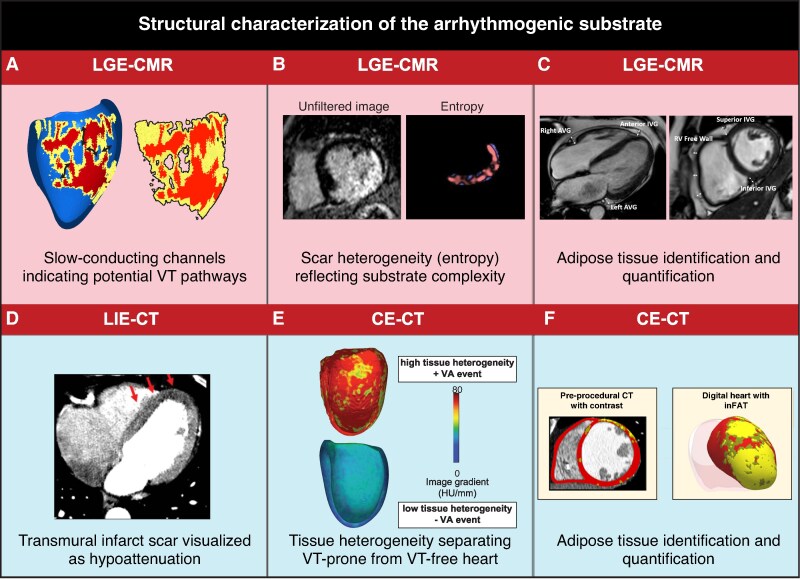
Advanced techniques for structural characterization of the arrhythmogenic substrate. (*A*) Thresholding of LGE-CMR provides a 3D model of slow-conducting channels and interface area: healthy myocardium (blue), border zone (yellow), and scar core (red) of the left ventricle, including slow conduction channels (black arrows), and the interface area between border zone and core scar.^18^ (*B*) Heterogeneity (entropy) of pixel signal intensities in LGE-CMR images, without prior thresholding.^24^ (*C*) LGE-CMR adipose tissue quantification at different locations (atrioventricular and interventricular grooves, right ventricle free wall).^22^ (*D*) Hypoattenuation of ischaemic area in dual-energy LIE-CT image.^25,26^ (*E*) Ce-CT tissue heterogeneity in ischaemic cardiomyopathy, showing a 3D model of the left ventricle in patients with high (arrhythmic) and low (arrhythmia-free) heterogeneity.^27^ (*F*) Ce-CT image and 3D model of the left ventricle divided into non-injured tissue (red), infiltrating adipose tissue (yellow), and fat–myocardium admixture (dark yellow).^28^  Ce, contrast enhanced; CMR, cardiac magnetic resonance imaging; CT, computed tomography; LGE, late gadolinium enhancement; LIE, late iodine enhancement.
